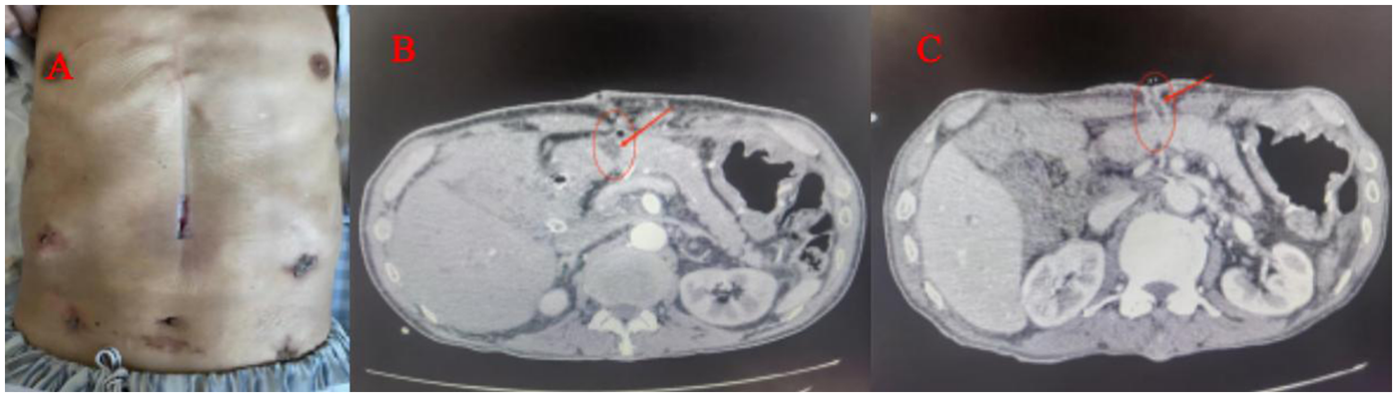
(A) POPF ensuing wound exudation pancreatic juice, which caused the skin around the wound redness and hot pain. (B) Contrast-enhanced CT shows fluid collections and a gap (arrows)of pancreaticojejunostomy. (C) When the amount of the wound exudation were less than 100 ml, repeat CT enhancement show that the effusion was reduced and a fistula (arrows) formed between the pancreatic anastomosis and the incision in the abdominal wall.
